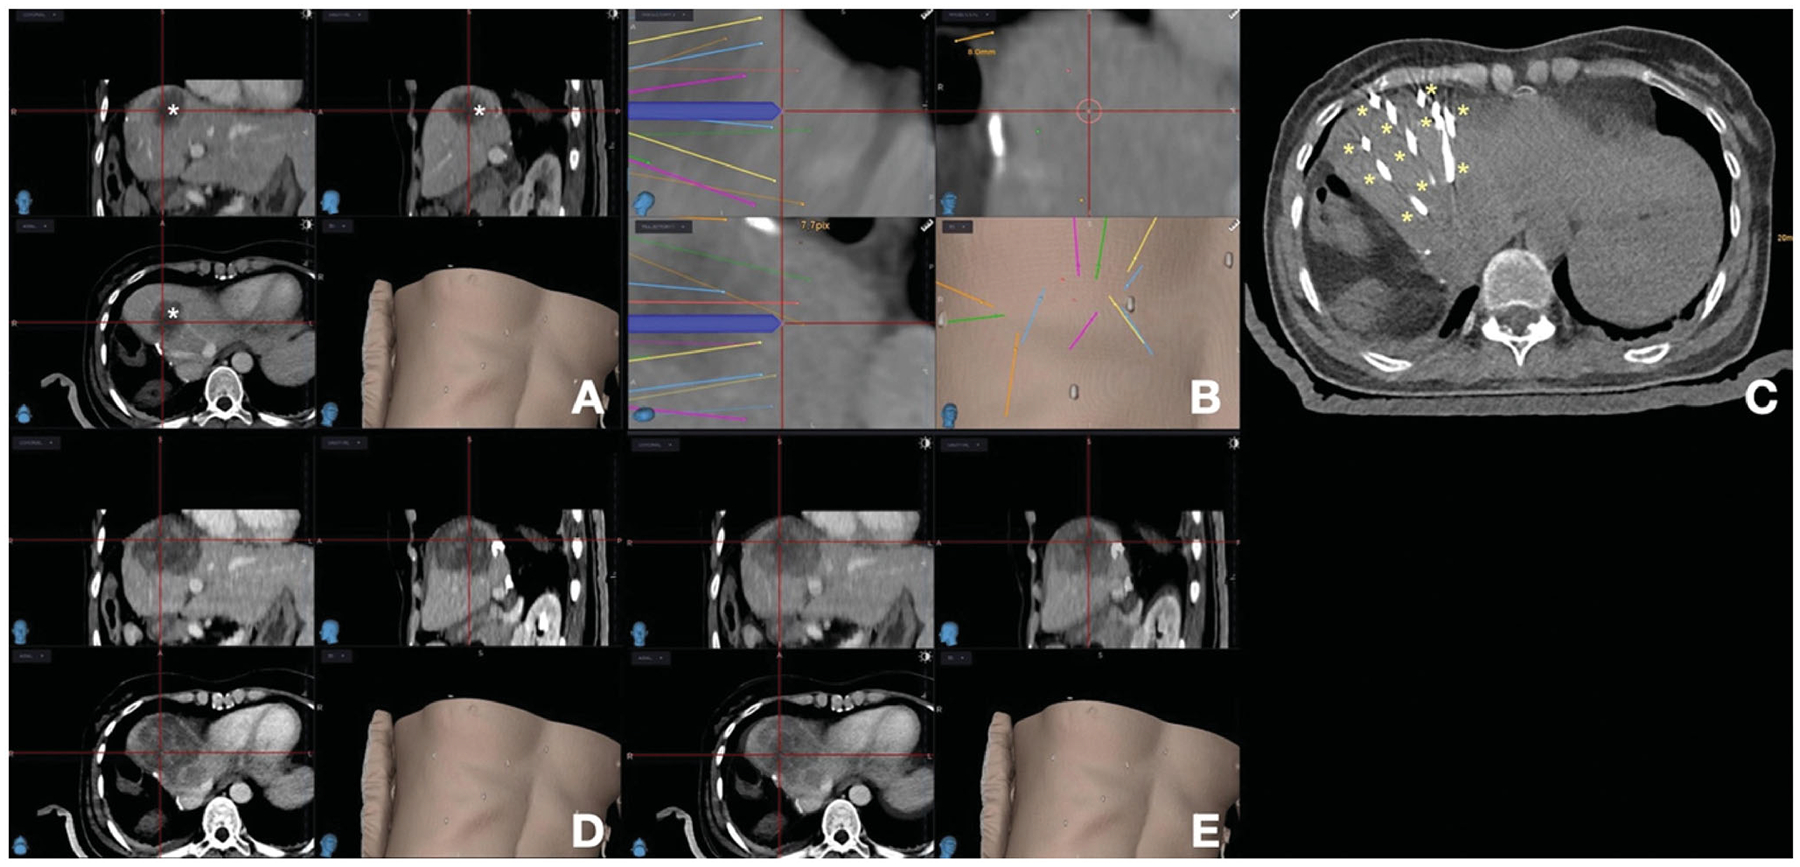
Stereotactic navigation systems on a patient with colorectal liver metastasis (CLM) undergoing liver ablation. (A) Posthepatectomy recurrent CLM measuring 5 cm in diameter (*); (B) Planning of 13 percutaneous guide needles trajectories, represented by different lines; (C) CT evaluation of guide needles placement (*); (D) Postablation CT for treatment verification. (E) Fusion of pre- and post-ablation CT for verification of ablation margin, showing complete tumor coverage with ablation with sufficient ablation margin.
